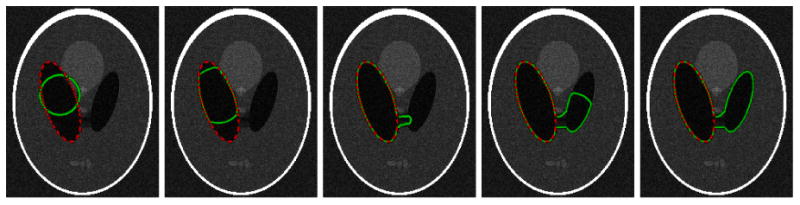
Desired segmentation and {*ϕ*(x, t) = 0} level-set overlayed on image *I*(x). A user would like to segment the “left ventricle” in this synthetic image that resembles an MRI scan of the brain. Evolving *ϕ* according to Eq-9 shrinks *E*(*t*) successfully (Fig4). However, the open-loop system fails to segment the desired region.
